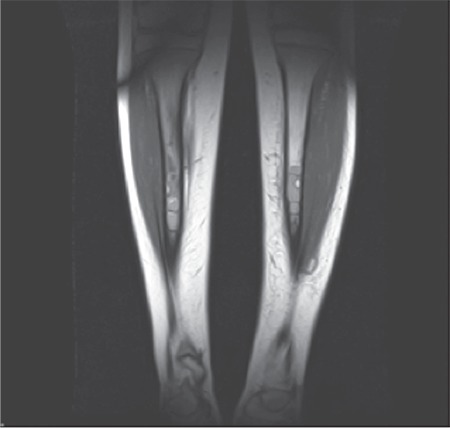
Magnetic resonance imaging of the lower extremities.
